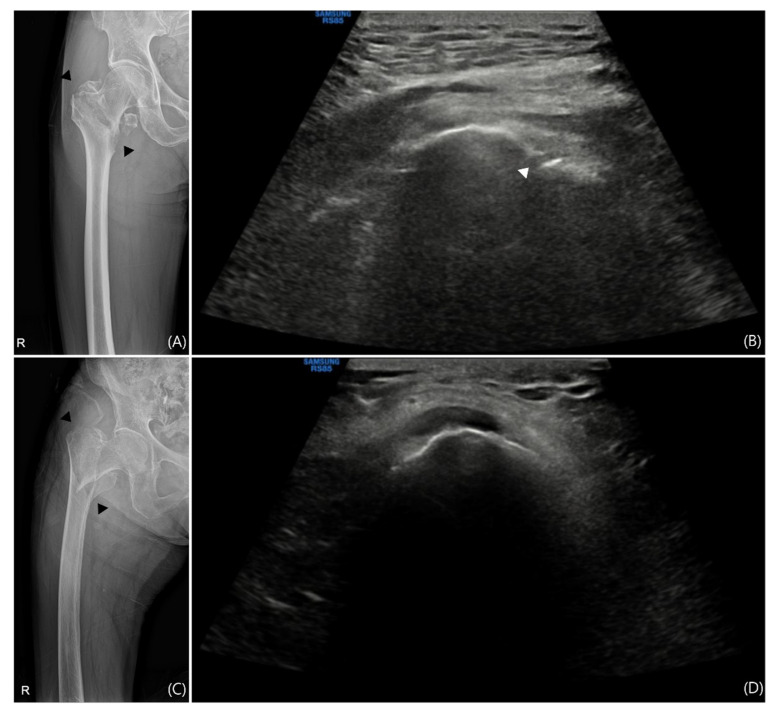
Comparison of X-ray and Ultrasound in Patients with Trochanteric Fracture. Not all trochanteric fractures are accompanied by greater trochanteric (GT) fractures. (A) The X-ray image of a patient with a trochanteric fracture and (B) the short-axis (SAX) view ultrasound image of the GT region from the same patient are shown. A GT fracture is visible on the X-ray and is also confirmed on sonography. If a tear is observed in this region, it is unlikely to have occurred iatrogenically. In another patient with a trochanteric fracture, (C) the X-ray image and (D) the SAX view ultrasound image show no evidence of a GT fracture on sonography. If a gluteal tendon tear is observed here, it suggests that the tear is of iatrogenic origin.
